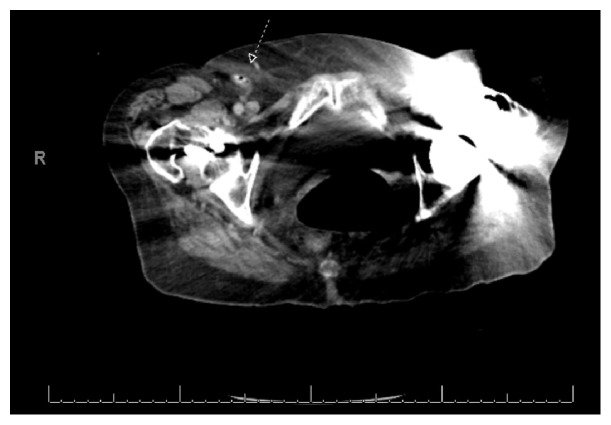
A second CT abdomen/pelvis image of Amyand's hernia is indicated by arrow.
